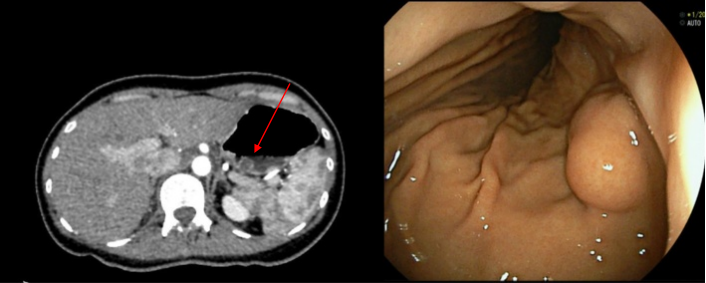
Abdominal computed tomography showing 2.5-cm submucosal tumor on the posterior wall of the gastric fornix with intra-gastric growth, and its endoscopic finding showing a distance of 4.0 cm between the lesion and the esophago-gastric junction
